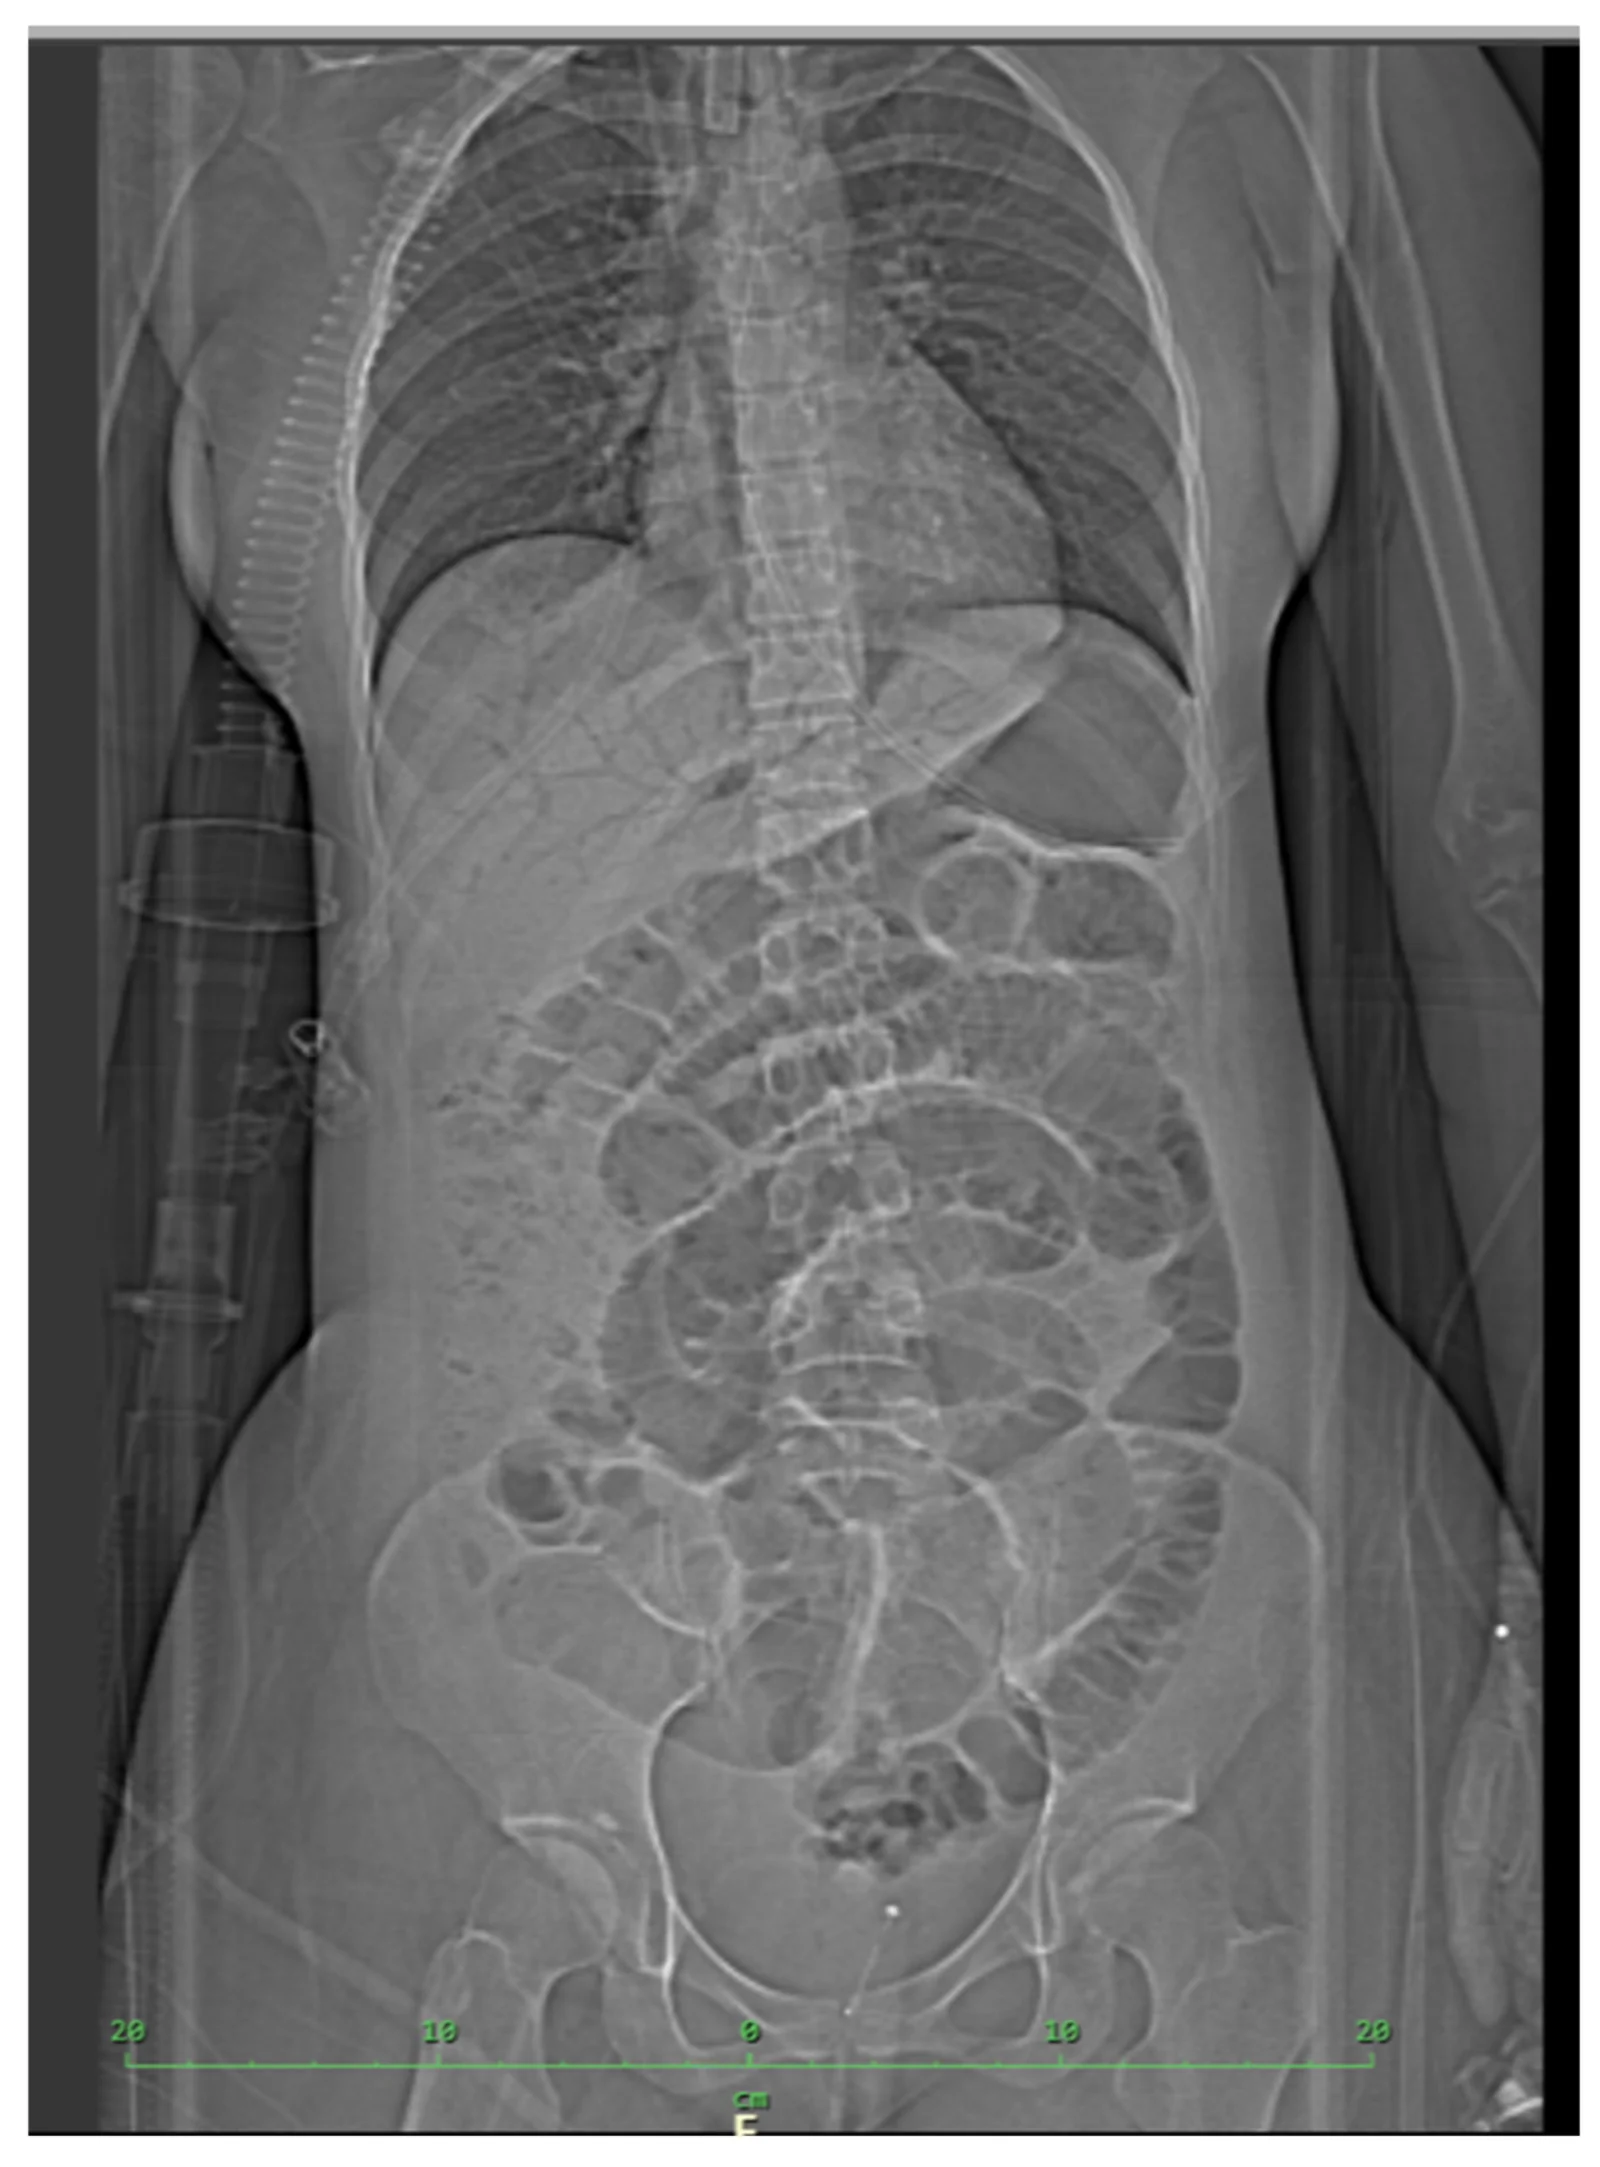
Abdominal CT imaging demonstrating extensive bowel distention in the acute phase of toxic epidermal necrolysis.
